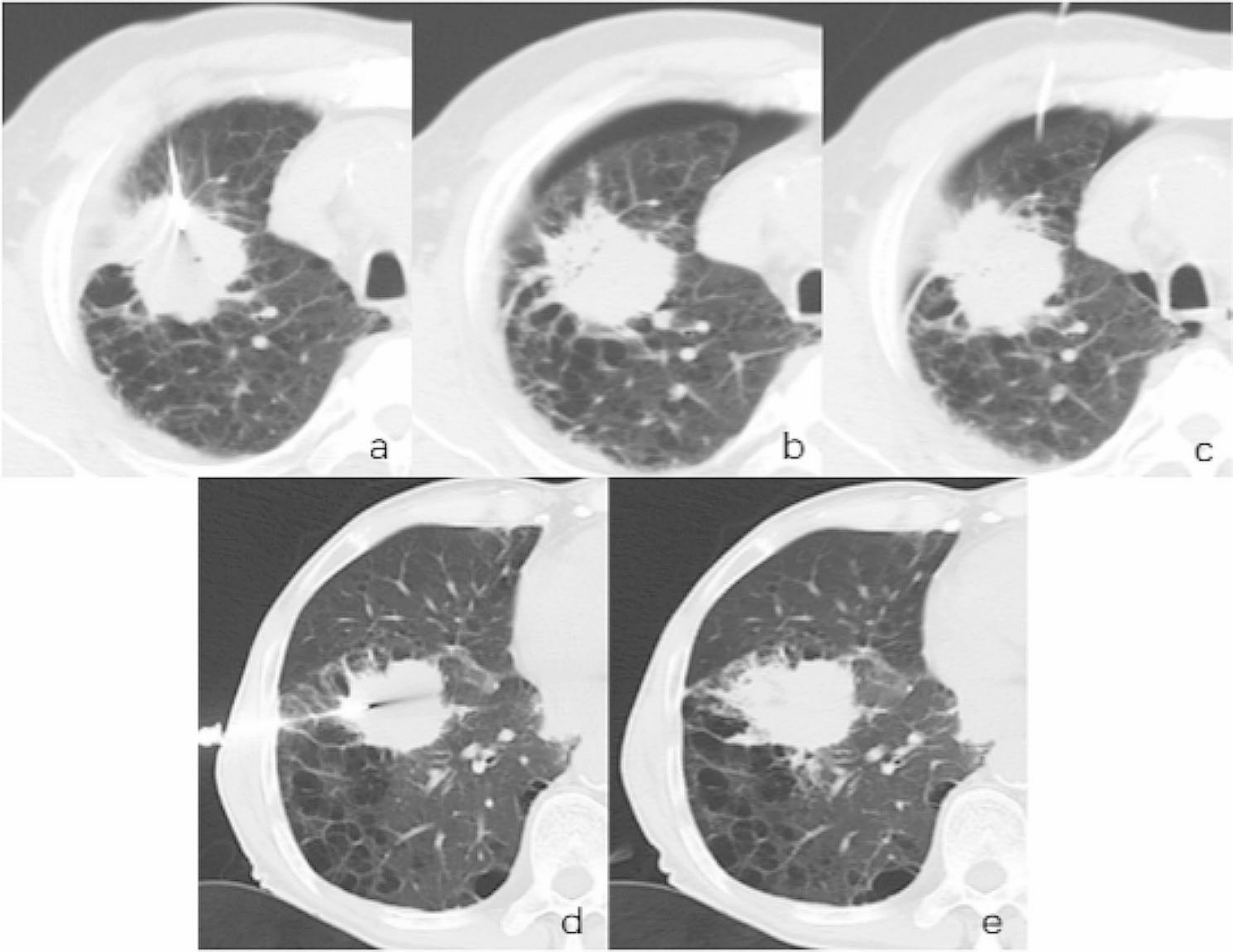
Unenhanced axial CT images (a) -(c) images of a patient (68Y/M) with grade e emphysema who did not undergo tract embolization during the procedure, and images (d) - (e) images of another patient (66Y/M) with grade e emphysema who underwent tract embolization during the procedure. (a) Image showing the insertion of the biopsy needle into the lung lesion. (b) Image showing the presence of major pneumothorax after the biopsy needle had been removed. (c) Image showing that the chest tube was placed and the gas inside the chest cavity was aspirated. (d) Image showing biopsy needle insertion into lung lesion. (e) Image showing that no pneumothorax occurred after the biopsy needle was removed. CT: computed tomography
